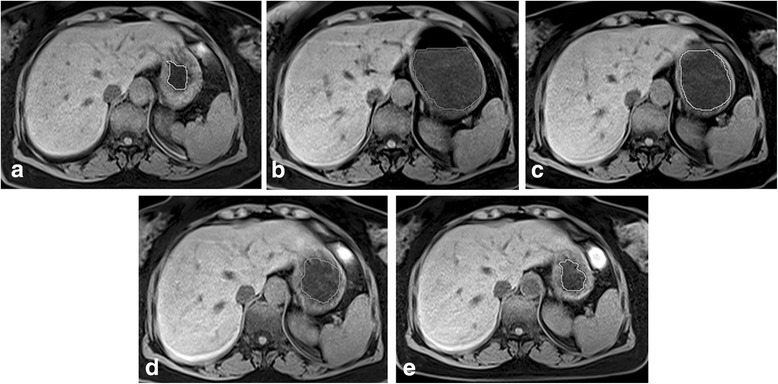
The participant underwent five serial epigastric MRI scans. The volume of fluid in the stomach was circled and calculated by AW suit software (GE company, USA) at the fasting state, 30 min, 60 min, 90 min, and 120 min after drinking carbohydrate solution separately (a–e)
